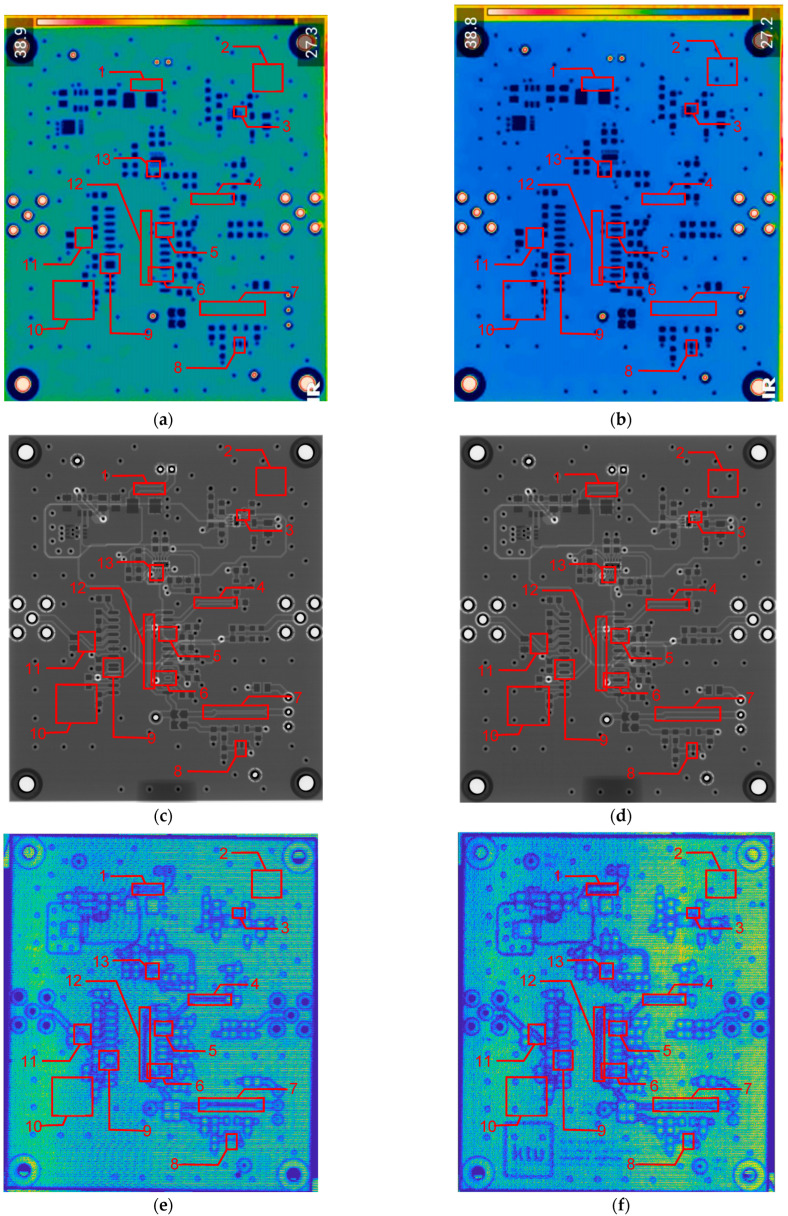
Comparison of images obtained by multiple NDT techniques of the good and defective PCBd produced using additive technology: (a) thermal image of the defective PCB; (b) thermal image of the good PCB; (c) radiographic image of the defective PCB; (d) radiographic image of the good PCB produced using additive manufacturing technology; (e) ultrasonic image of the defective PCB; (f) ultrasonic image of the good PCB produced using additive manufacturing technology. Explanation of the numbered zones are given in Table 1.
